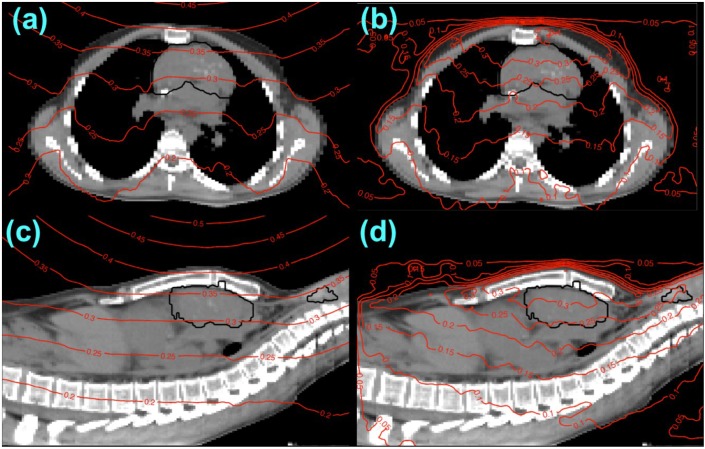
Axial (a) and (b) and sagittal (c) and (d) planes of equivalent neutron dose overlaying CT images of the HL patient, with dose calculated using the analytical *H/D* model (a) and (c) and the Monte Carlo *H/D* model (b) and (d). Equivalent dose values are percentages of the prescribed target dose. The mediastinal target volume and thyroid are indicated by black contours.
